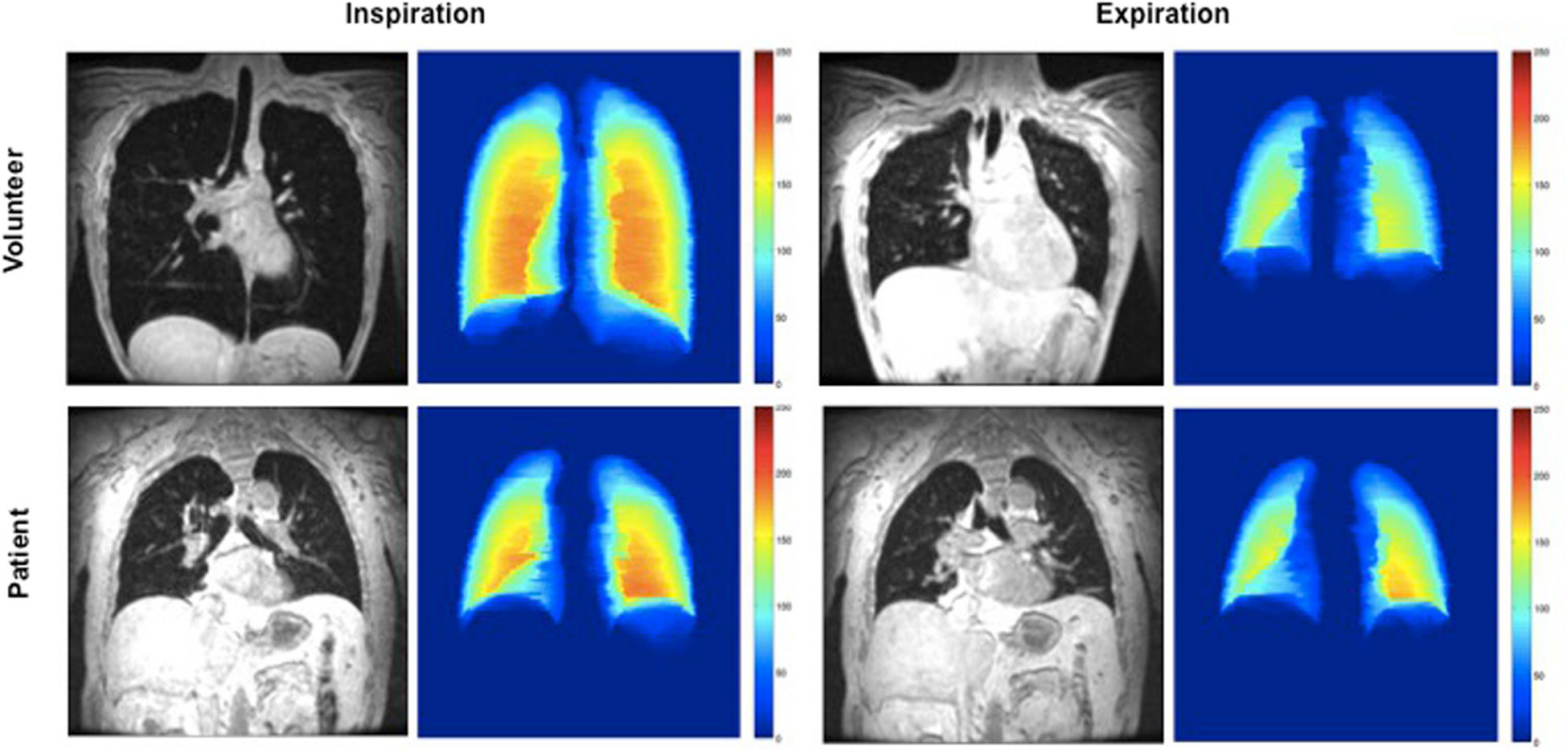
MR images and color maps at maximum inspiration and expiration MR images during 12-second breath-holds in inspiration and expiration in a patient with Pompe disease and a healthy volunteer. The color maps represent the thickness of the segmentation in the anterior-posterior axis (red being the thickest and blue being the thinnest). Note the limited increase in vertical length in the Pompe patient relative to the increase in the healthy volunteer.
